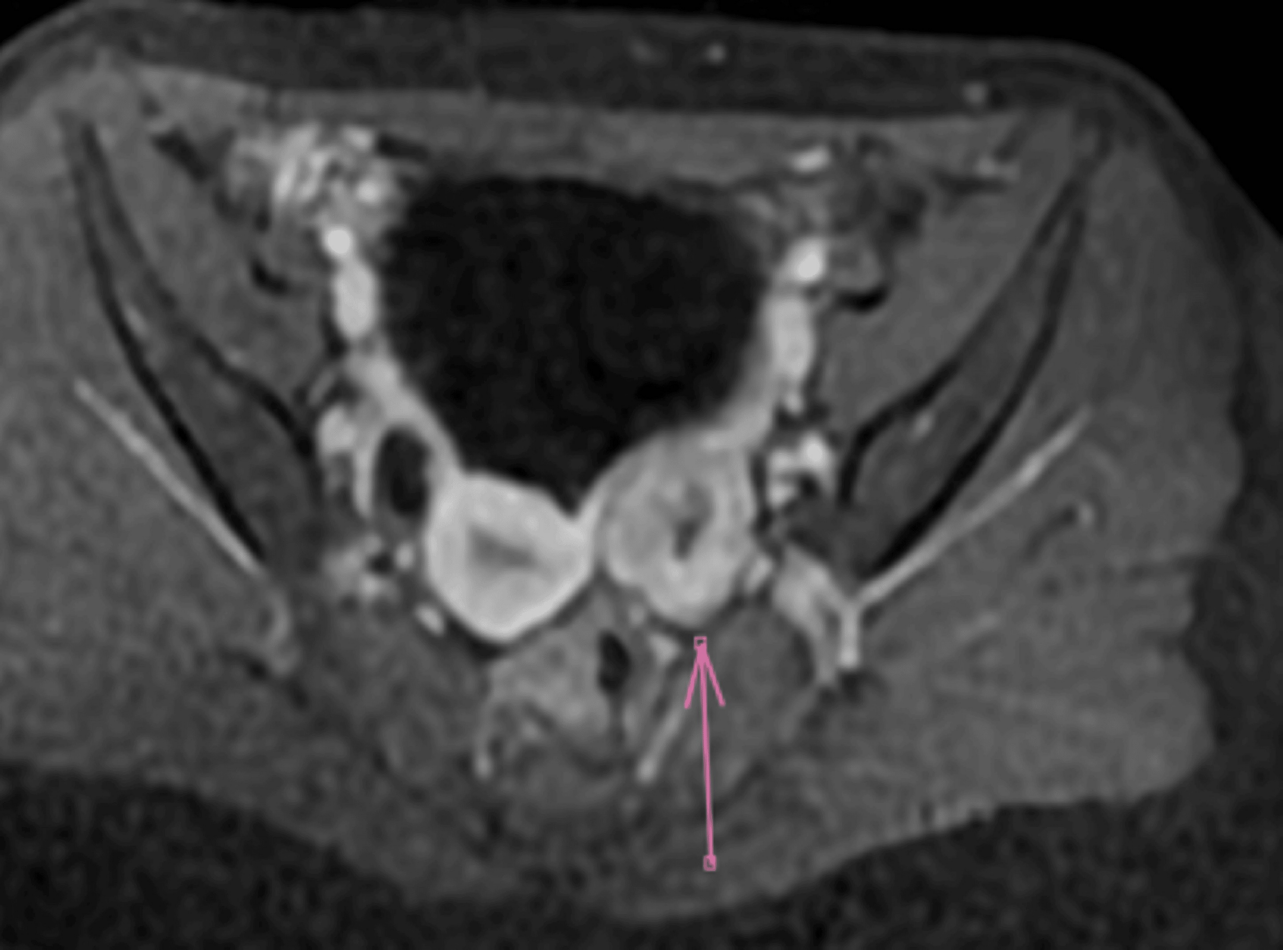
Axial pelvic MRI in T2-weighted imaging after gadolinium injection showing the presence of a mass classified as O-RADS 4 (arrow), highly suspicious for malignancy.
